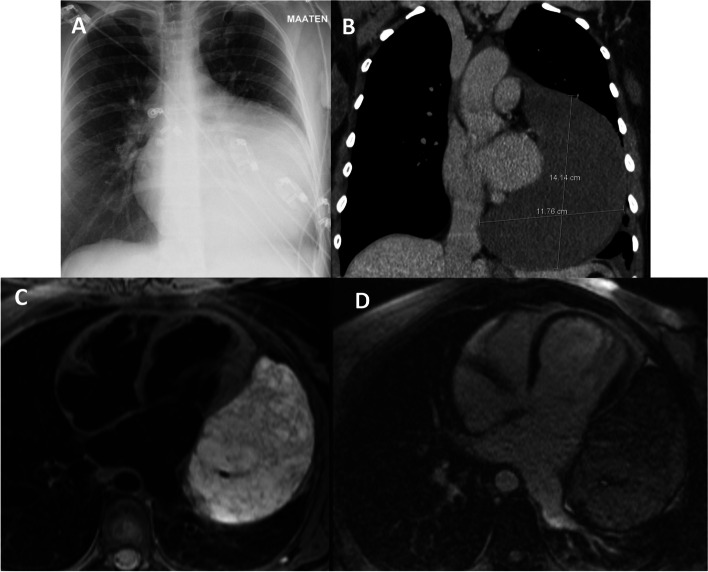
Imaging findings. A Chest X-ray showed an enlarged cardiac shadow without pneumothorax. B 14 cm diameter cystic pericardial tumor without calcifications, fat or contrast enhancement in CT. Both coronary and CT angiography demonstrated feeding arteries to the tumour from distal branches of LCX and RCA and venous drainage to coronary sinus. C T2-weighted image in CMR. D T1 weighted late enhancement image after contrast in CMR
